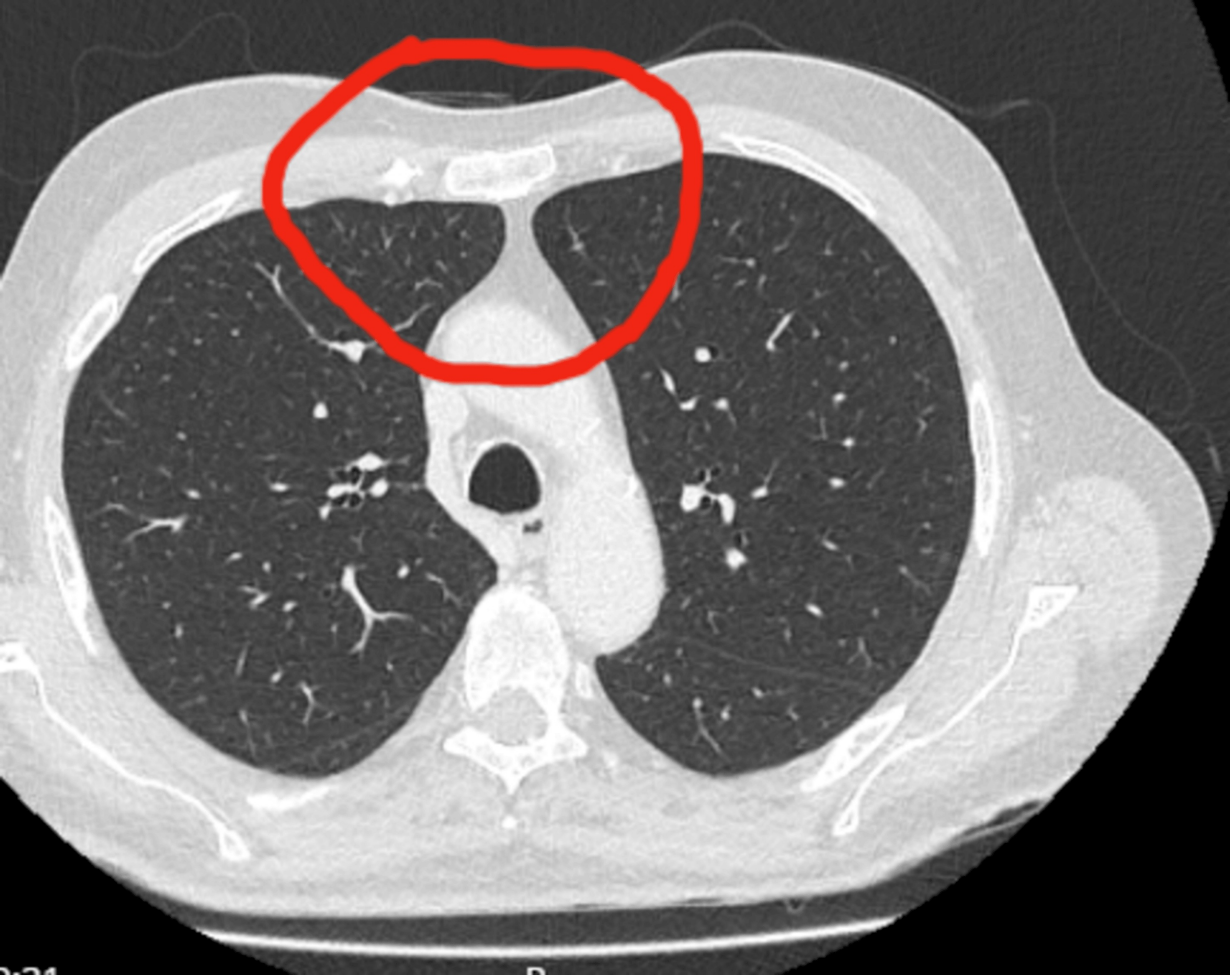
Axial CT scan of the chest An axial CT scan of the chest that does not show enlargement of the thymus gland (area marked in red).
